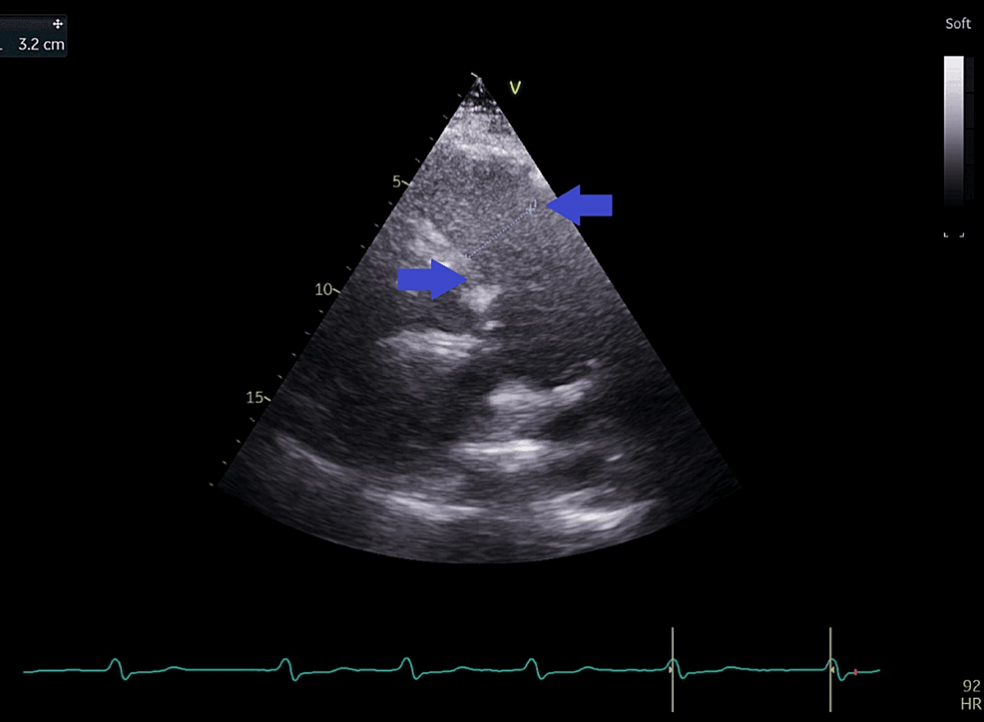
Left parasternal short-axis view at the base of the heart showing a moderately dilated pulmonary artery (blue arrows).
